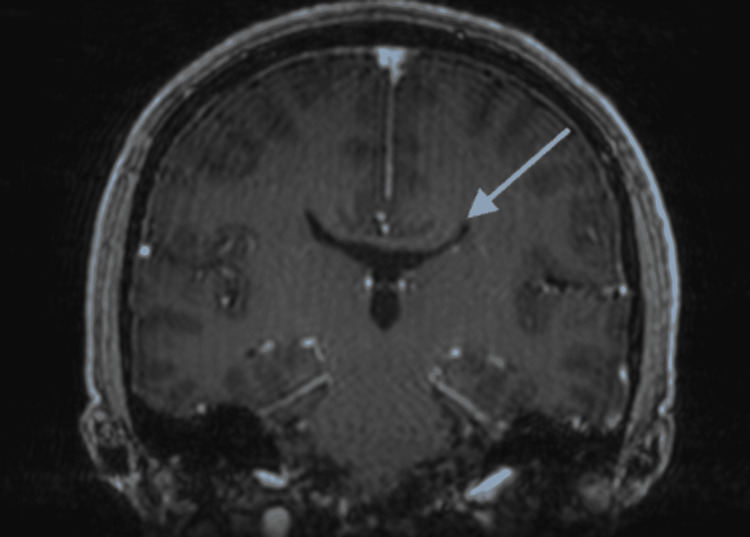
MRI head demonstrating Viking's helmet appearance of the lateral ventricles
